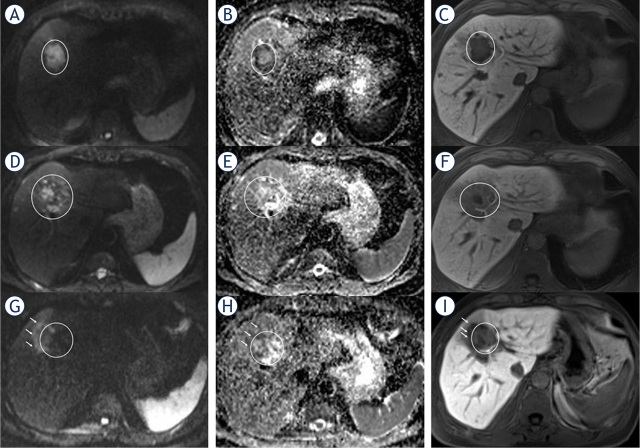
Non-responding metastases (NR-met) in a 56-year-old male. In preinterventional MRI, metastasis (circle) shows restricted diffusion (A+B) with an mean apparent diffusion coefficient (ADCmean) of 0.86 x 10^−3^ mm^2^/s and a hypointense pattern on the liver-specific phase (C). Three months after high-dose-rate brachytherapy (HDR-BT), the metastasis showed visually partial restricted diffusion (D+E), but, with an increasing ADCmean of 1.52 x 10^−3^ mm^2^/s and hypointensity in the hepatobiliary phase (F). After 11 months, the lesion increased in size, shows a visually an increasing diffusion restriction (K+L) at the boarder (arrow) with a persistently ADCmean value of 1.53 x 10^−3^ mm^2^/s and a new defect in the hepatobiliary phase (arrow) (I) indicating local tumor recurrence.
